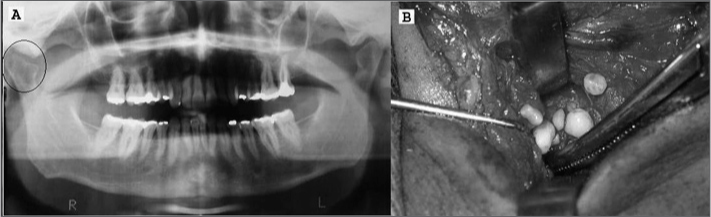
Panoramic mandible x-ray showing an alteration (Circle) in the right TMJ (A) and surgical removal of the synovial chondromatosis (B).
